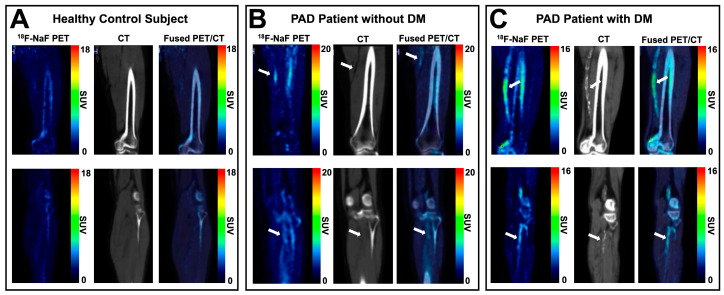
Examples of ^18^F-NaF PET/CT imaging in (A) a healthy control subject, (B) a PAD patient without diabetes mellitus (DM), and (C) a PAD patient with DM, demonstrating increased retention of ^18^F-NaF and increased arterial calcification in lower extremity arteries in the presence of DM and worsening status of PAD [46]. White arrows denote regions of arterial retention of ^18^F-NaF.
